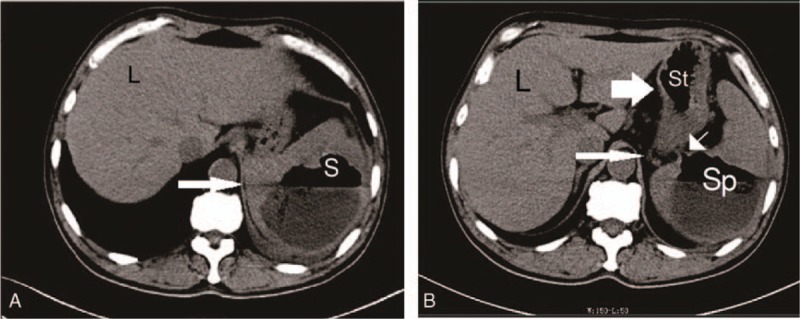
(A) In the CT Scan upon first admission, the white arrow shows the distinct air-fluid level seen within the spleen. L: Liver; S: Spleen. (B) Splenocolic fistula (small arrow) and its relation with the colon (thin white arrow) and stomach (thick white arrow). L: Liver; Sp: Spleen; St: Stomach.
